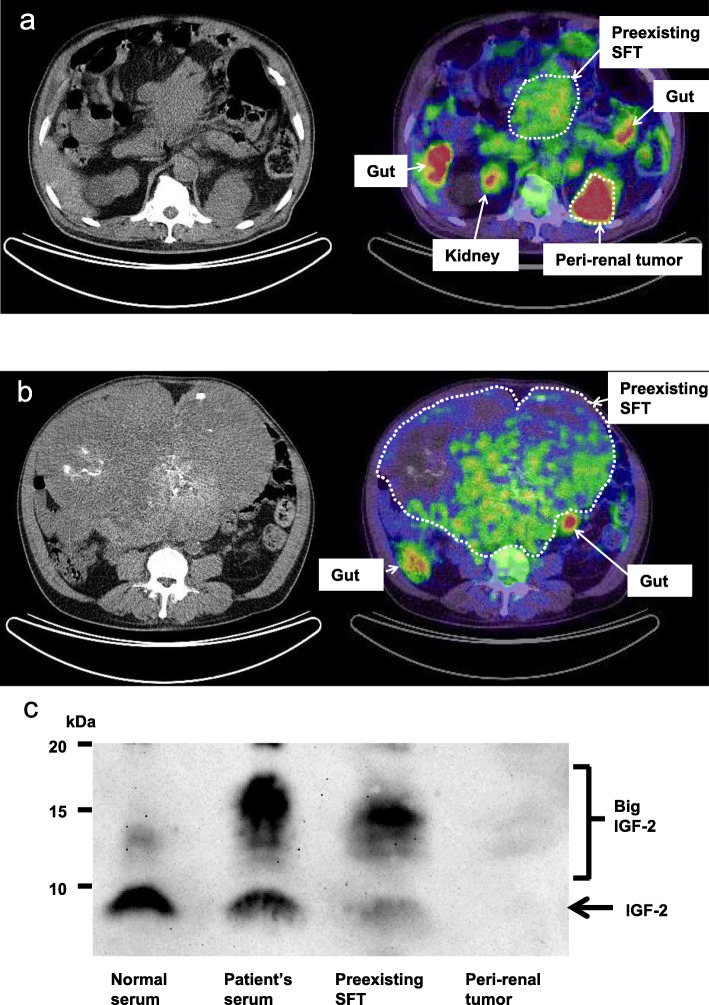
^18^F-FDG PET-CT scans of the tumors (a-b) and western immunoblot analysis with anti-IGF-2 antibody (c). ^18^F-FDG PET-CT scans obtained at the Th12 vertebral level (a) and at the L3 vertebral level (b) 15 months after the onset of the hypoglycemic episodes. Western immunoblot analysis with anti-IGF-2 antibody in the serum of a healthy subject (c, 1st lane), in the patient’s serum 18 months after the onset of hypoglycemic episodes (c, 2nd lane) and in the autopsy tumor samples (30 μg of total protein) of the preexisting SFT (c, 3rd lane) and the peri-renal tumor (c, 4th lane). The uncropped immunoblot image and details of the procedure including the information on anti-IGF-2 antibody are presented in Additional files 1 and 2, respectively. FDG: Fluorodeoxyglucose, PET-CT: positron emission tomography-computed tomography, IGF: insulin-like growth factor, SFT: solitary fibrous tumor
